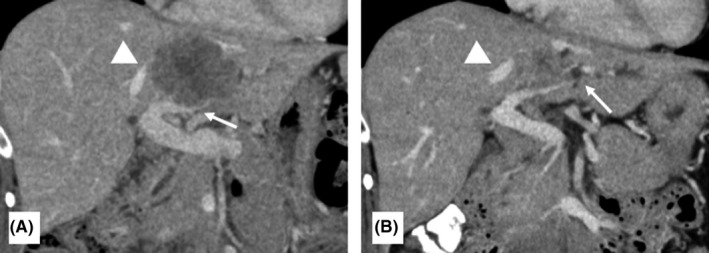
A, B, Contrast‐enhanced CT before first hepatectomy for colorectal liver metastases. A, Before neoadjuvant chemotherapy. Metastatic tumor in segment 2 &4 was involved the left glissonean pedicel (white arrow) and the main trunk of the middle hepatic vein (arrow head). B, After neoadjuvant chemotherapy. Metastatic tumor decreased the size, however, involvement of the left glissonean pedicle remained and the intrahepatic bile ducts in the segment 2 was dilated (white arrow). The tumor was still close to the main trunk of the middle hepatic vein (arrow head)
